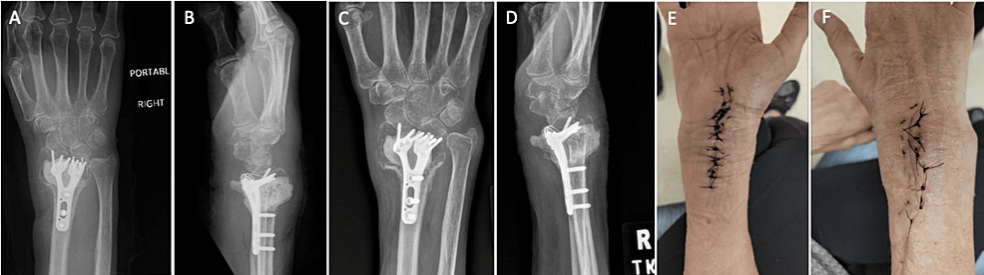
Postoperative X-rays and follow-ups. A-B) immediate postoperative anteroposterior and lateral X-rays; C-D) four-week postoperative anteroposterior and lateral X-ray views of the right wrist: hardware and cement appearing intact. There is residual loss of radial height radial, tilt is neutral, with unchanged bone resorption and lucency at the cement bone interface. Cement extends dorsally and volarly due to cortical breach. E-F) Volar and dorsal right wrist incisions with sutures in place at three weeks postoperatively.
